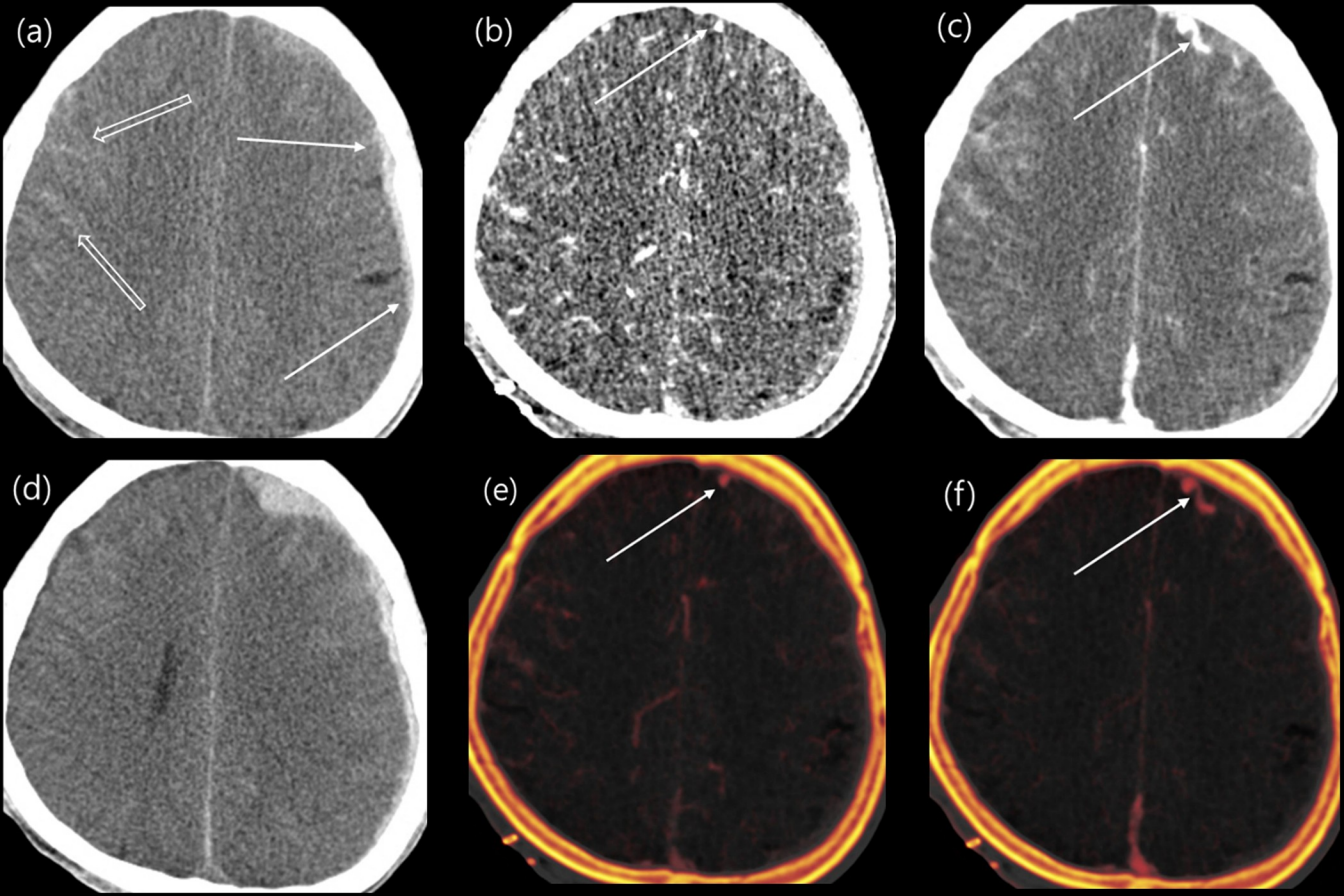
A representative case. A 50-year-old male patient visited our emergency department with a headache that occurred 30 min after a slip down before admission. On the initial precontrast brain CT (a), a thin acute subdural hematoma (SDH) was observed in the left frontal convexity (arrows), and an acute subarachnoid hemorrhage (open arrows) was observed along the right frontal cortical sulci. CT angiography was performed because of the possibility of cerebrovascular injury. Growing contrast leakage was observed in the arterial and venous phases of dual-energy CT (b, c, e, f). It was observed on both the fusion (e, f) and conventional-like images (b, c) but was clearly confirmed on the fusion images. On follow-up CT after 5 h (d), the amount of left frontal SDH was significantly increased.
